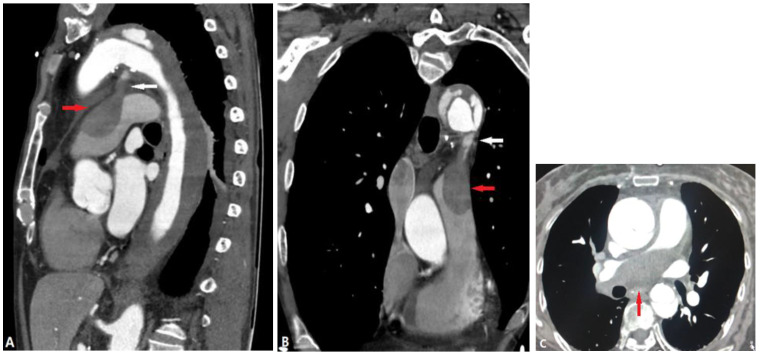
Aortic dissection. (A,B): Sagittal and coronal CTA images show the hematoma of an aortic dissection extending to the pulmonary artery via the PDA (white arrow), which causes PA stenosis (red arrow). (C): Another aortic dissection patient presents with right pulmonary artery stenosis (red arrow) because of the discontinuity of the media. CTA—computed tomography angiography; PDA—patent ductus arteriosus.
